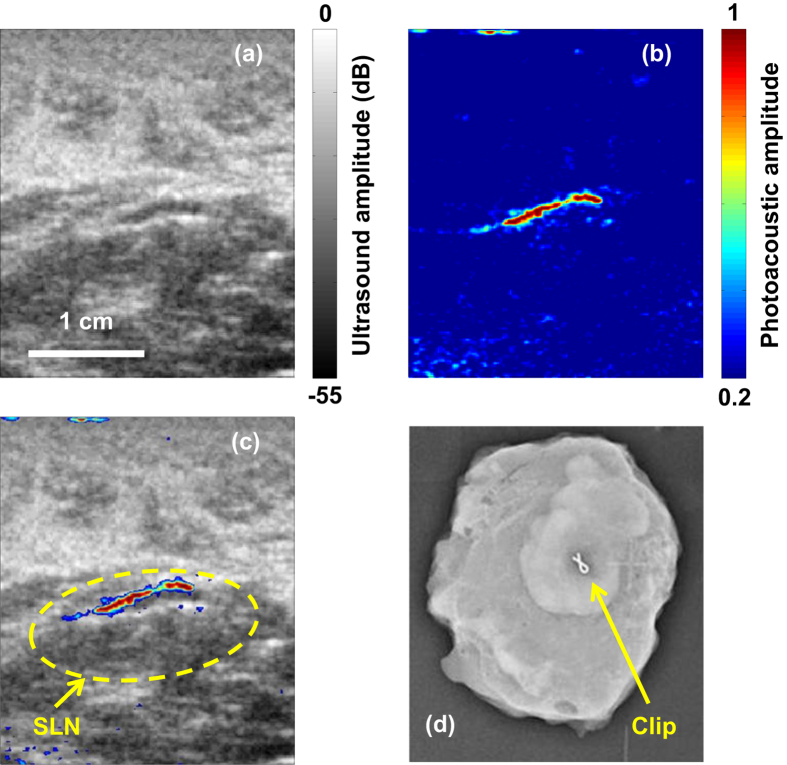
(a) *In vivo* US image of a lymph node. (b) *In vivo* PAT image of methylene blue dye. (c) Co-registered PAT-US image of the SLN. (d) Radiograph of *ex vivo* SLN showing the presence of the tissue marking clip.
